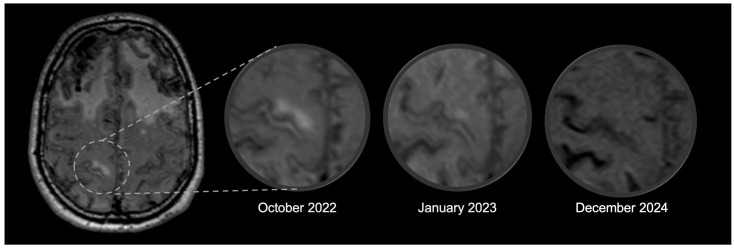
Spontaneous area of vasogenic edema (ARIA-E) in an iCAA patient. The alterations in the right precentral gyrus visible in FLAIR gradually resolved following immunosuppression. Legend: ARIA = Amyloid-related Imaging Abnormalities; iCAA = iatrogenic Cerebral Amyloid Angiopathy; E = edema/effusion.
